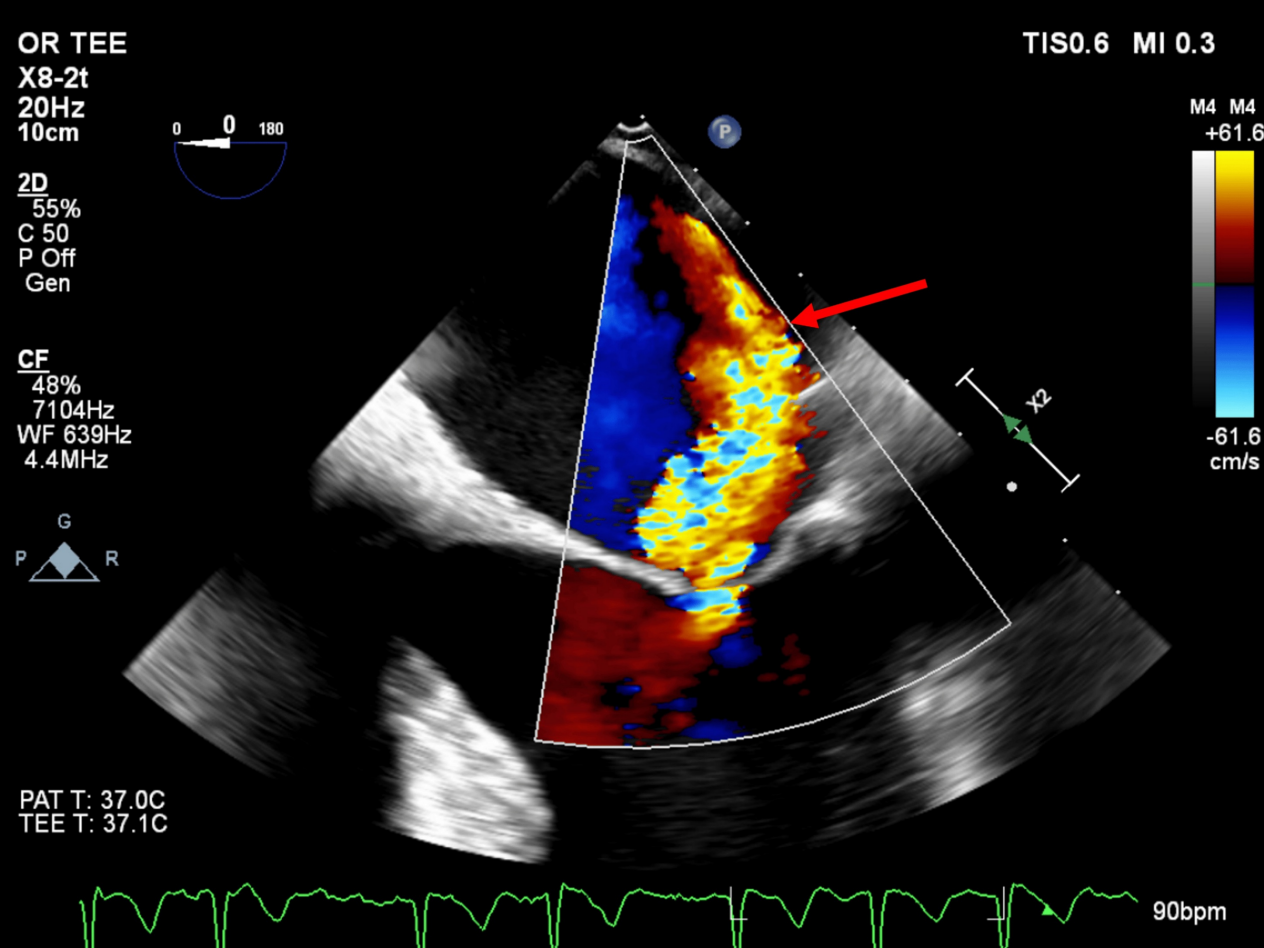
Eccentric component of mitral regurgitation jet. Midesophageal 4-chamber view obtained with transesophageal echocardiography showing the eccentric component of the mitral regurgitation jet (arrow) with associated Coanda effect. The Coanda effect refers to when an eccentric jet is drawn towards the chamber wall and appears to cling to it (in this case, the left atrial wall). These jets are prone to underestimation of severity by jet area using color flow Doppler [6].
